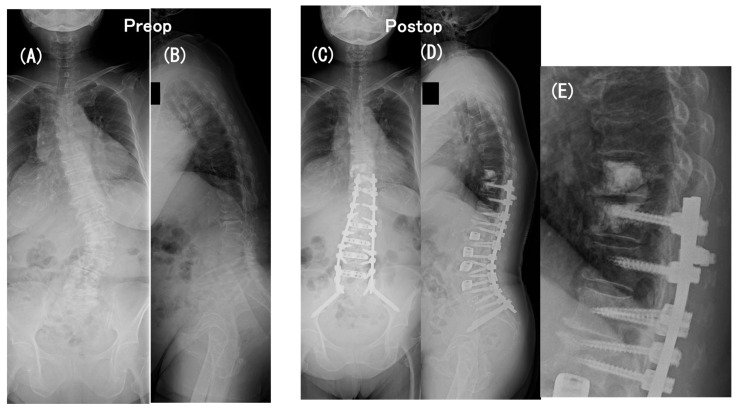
A 78-year-old woman with persistent low-back pain and loss of ambulatory endurance with scoliosis and severe sagittal spinopelvic imbalance. Coronal (A) and sagittal (B) full-length standing radiographs obtained preoperatively revealed the following: coronal Cobb angle = 42°, LL = 19°, PI = 56°, PI–LL = 37°, PT = 31°, TK = 18°, and SVA = 167 mm. Coronal (C) and sagittal (D) full-length standing radiographs and a close-up lateral radiograph (E) obtained at an early postoperative period. A two-stage operation, one week apart, comprised (1) the first-stage operation for the LLIF procedure at L1-2 through L4-5 with the patient in the lateral position and (2) the second-stage operation for posterior instrumentation with the patient placed prone, consisting of PPS fixation at L1 through S1, mini-open transforaminal lumbar interbody fusion (TLIF) at L5-S1 with S2-alar-iliac screw fixation, and a traditional open approach for PS fixation and fusion at T10 through T12 with prophylactic PMMA cement augmentation at T9 and T10. Postoperatively, the coronal Cobb angle decreased to 7° and sagittal spinopelvic parameters improved to 53° in LL, to 2° in PI–LL, to 16° in PT, to 46° in TK, and to 82 mm in SVA.
